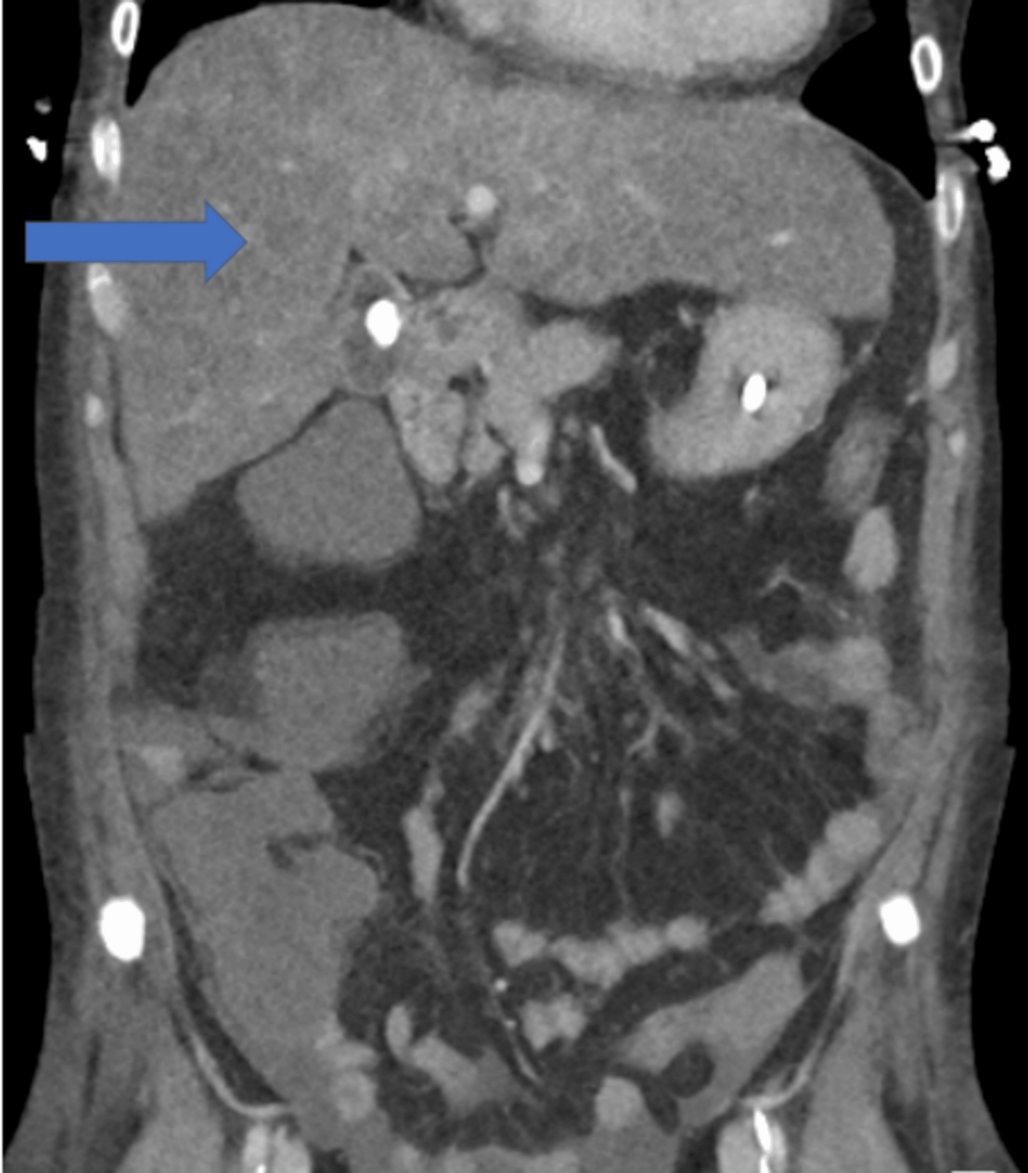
CT abdomen and pelvis (coronal section) undertaken on day 6 revealing the state of the liver (fatty liver changes with nodular margins)
